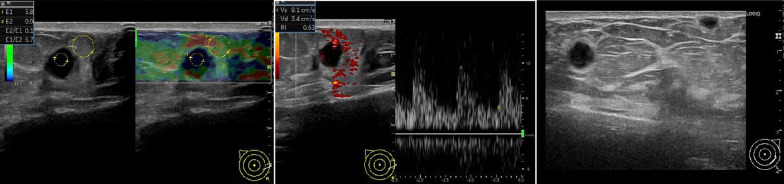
Ultrasonography revealed multiple round, well-defined, full, hypoechoic masses with internal pulsatile blood flow signal in both breasts. Elastography showed relatively uniform firmness
